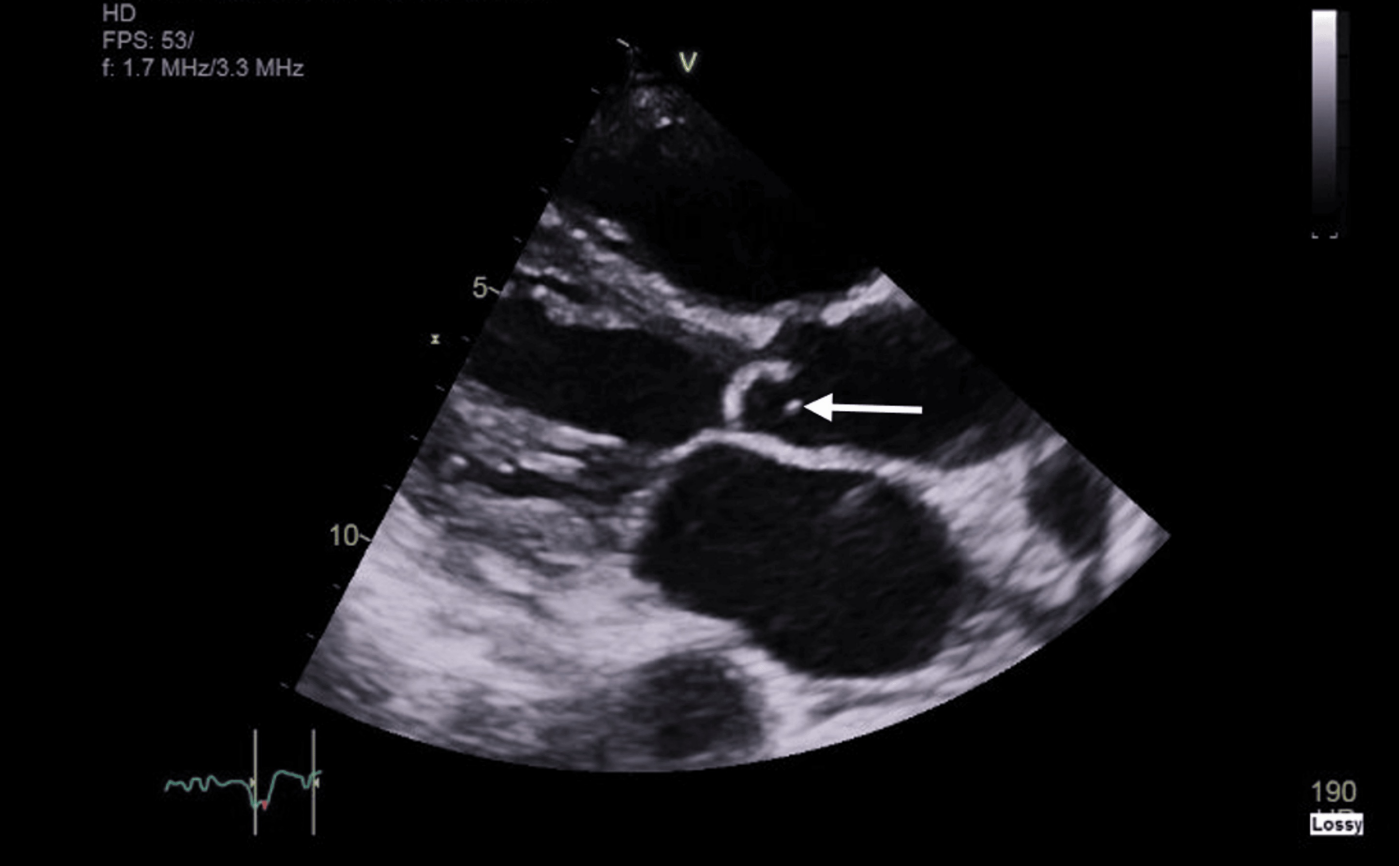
Transthoracic echocardiogram (TTE) revealing an echogenic mobile structure in the supravalvular region of the aortic valve (indicated by the arrow).
